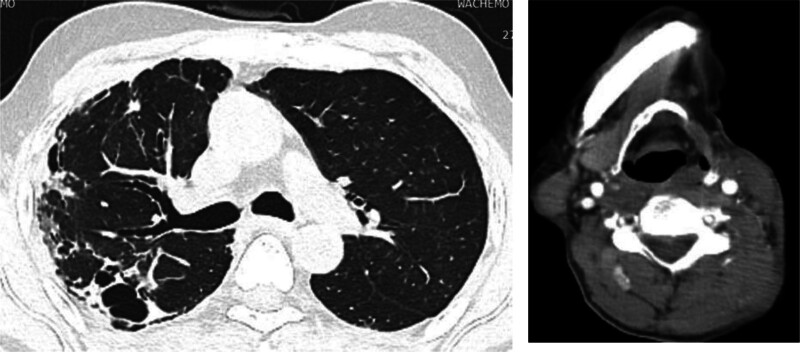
Follow-up neck and chest CT with contrast (done on 12 wk of treatment initiation) shows right upper lobe fibro bronchiectasis likely post tuberculosis with resolution of left internal jugular vein thrombosis. CT = computed tomography.
